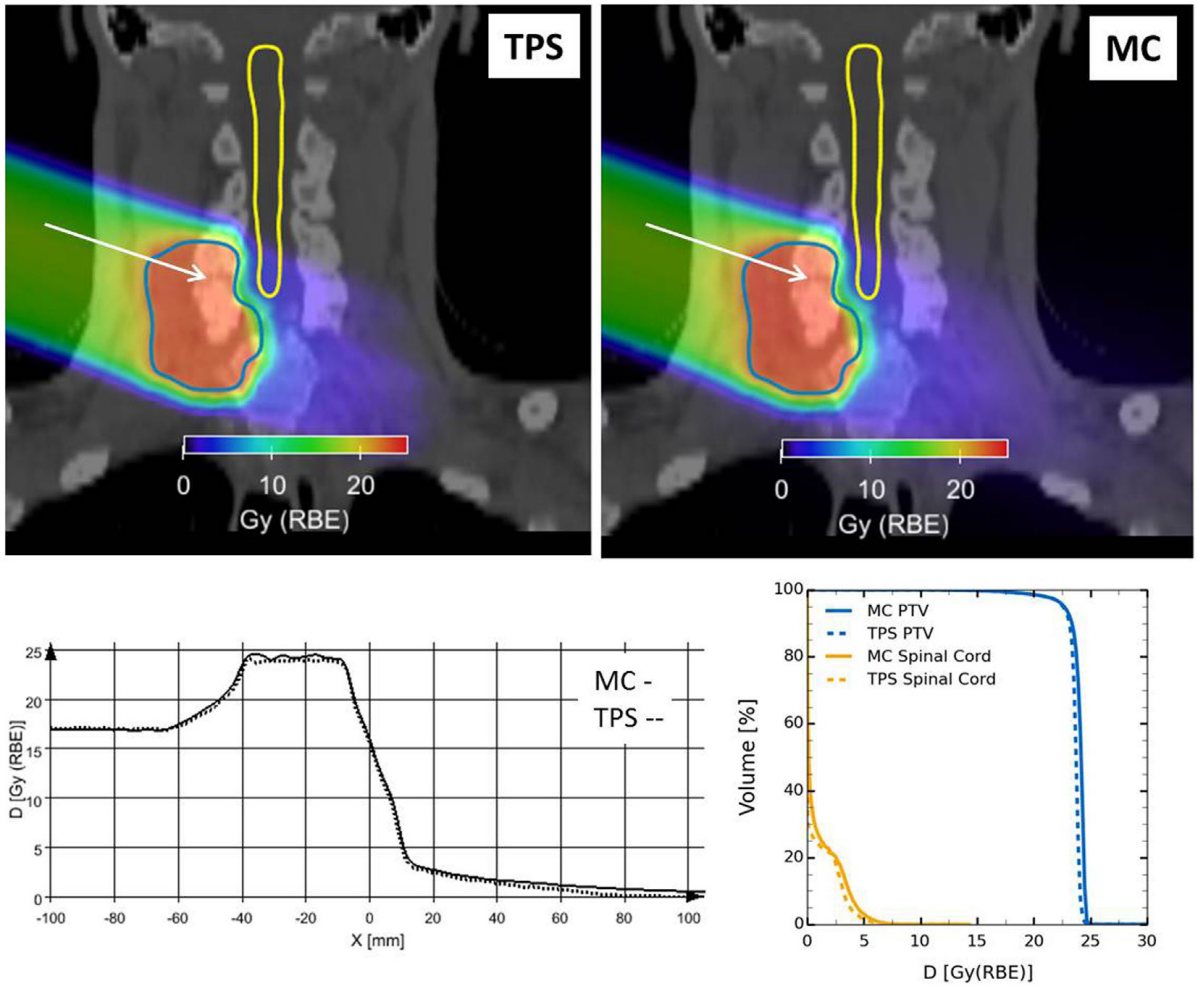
Clinical-like carbon ion therapy plan delivered to the upper spine region in a single right-lateral field. Top: overlay views of RBE-weighted dose distributions obtained from the TPS (left) and the FLUKA framework (right) on the treatment planning CT image. Outlined are the PTV and the spinal cord. Bottom: comparison of TPS and MC RBE-weighted dose profiles along the white arrow drawn in the overlay views (left); dose volume histograms for PTV and spinal cord for both TPS and MC RBE-weighted dose distributions (right).
